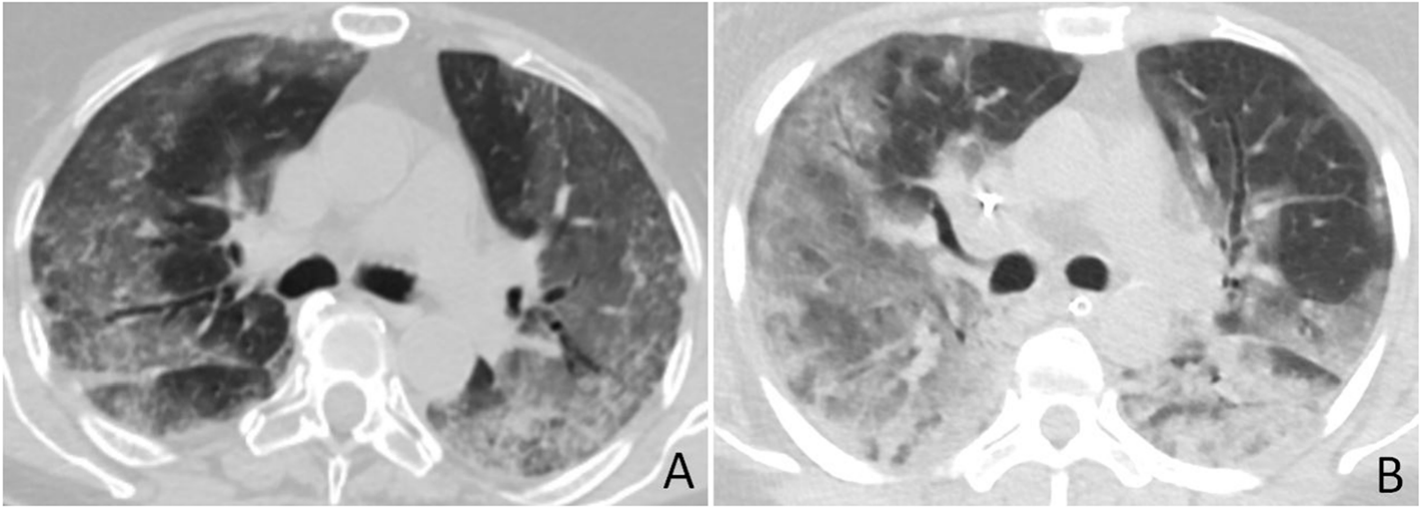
COVID-19 and MERS pneumonia misdiagnosed as COVID-19. a A 69-year-old man with positive PCR test for COVID-19; axial HRCT image shows peripheral and central GGO with superimposed interlobular septal thickening, bronchial dilatation, and pleural thickening. b Axial CT image of a 50-year-old man with a positive PCR for MERS shows similar findings as a with posteriorly located consolidations in the right lung
